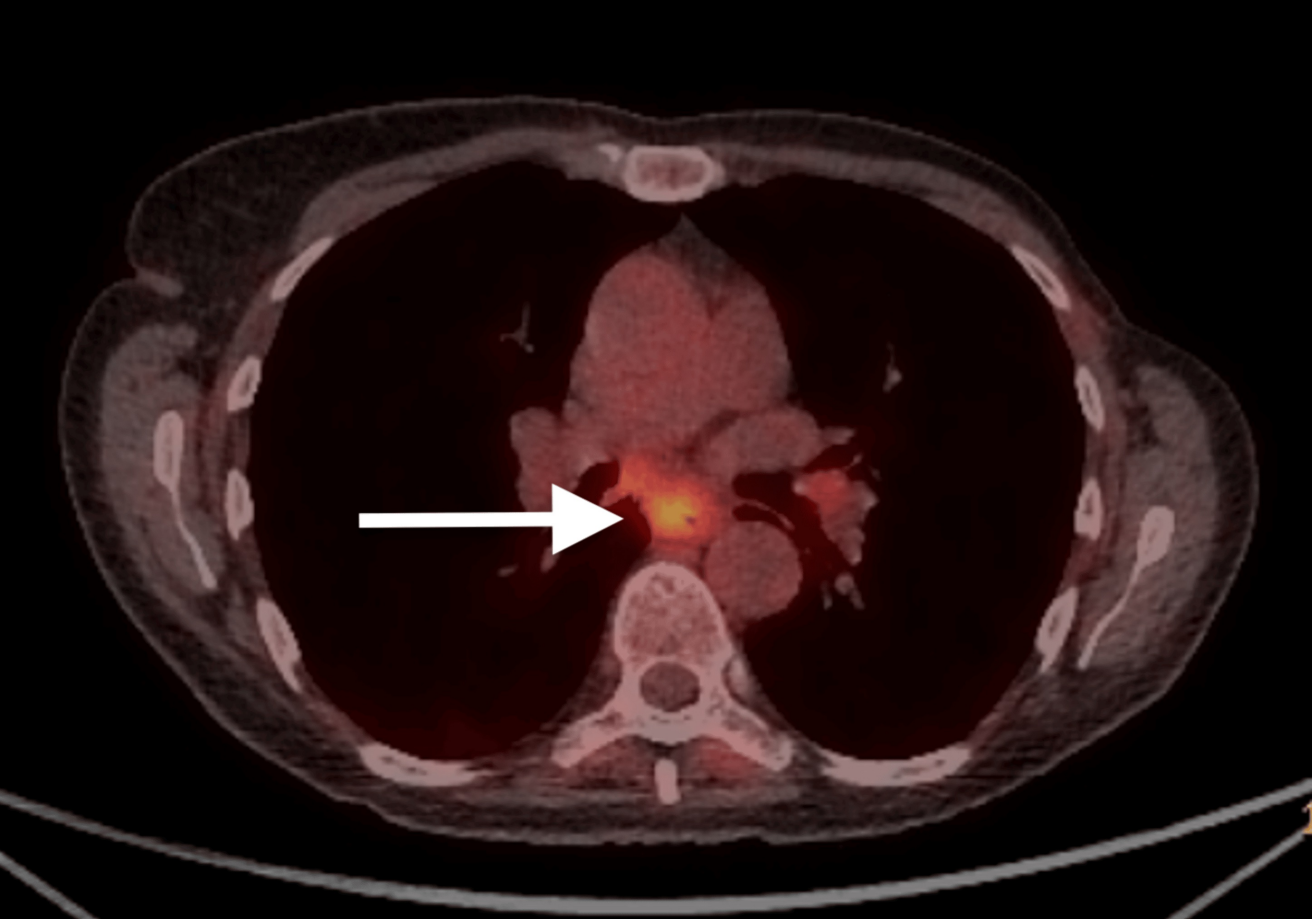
PET scan demonstrating soft tissue infiltration at the level of the carina, which was inseparable from the esophagus, with high FDG uptake (white arrow). PET: positron emission tomography, FDG: fluorodeoxyglucose.
